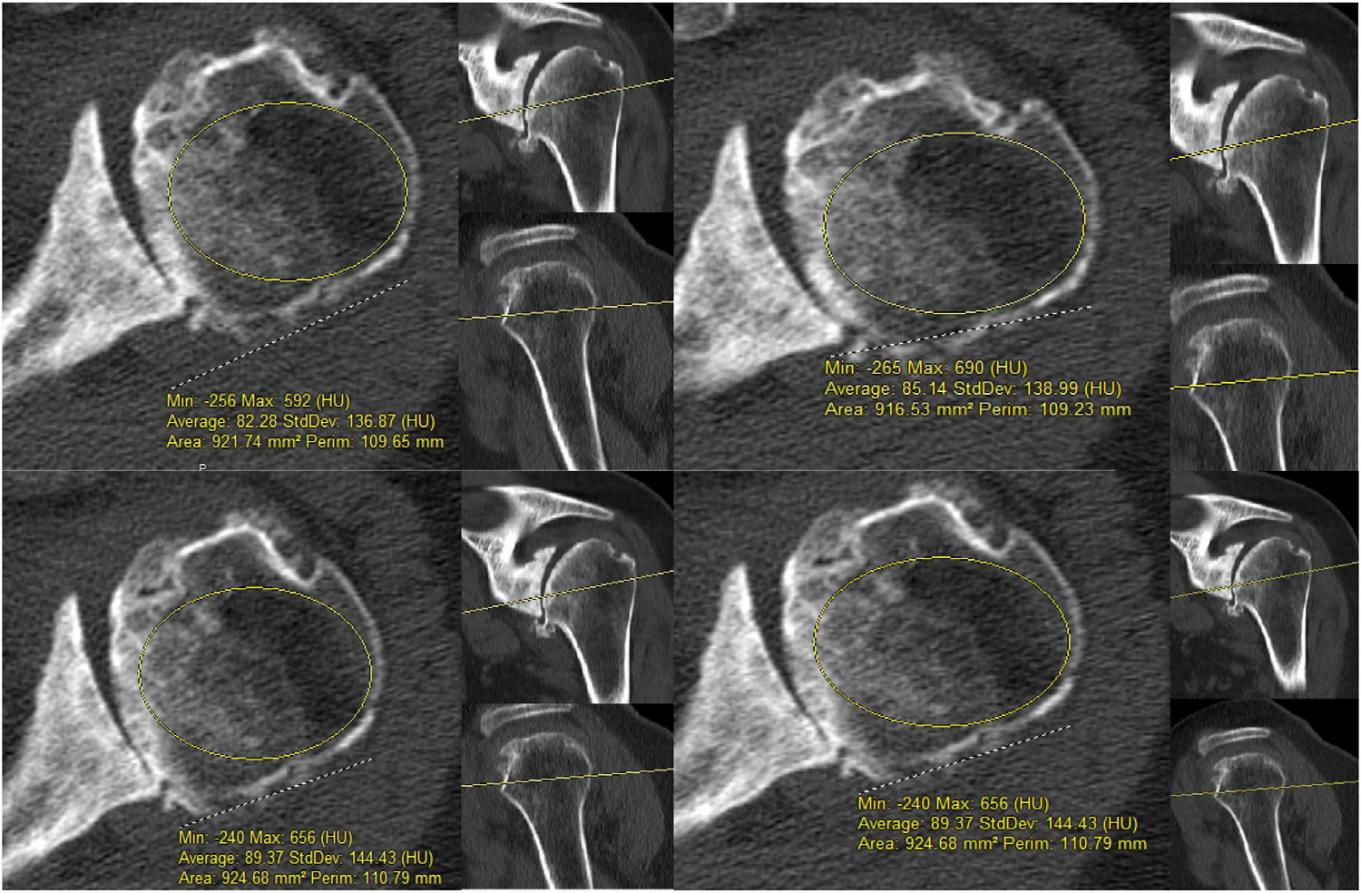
Computed tomography (CT) Hounsfield unit (HU) measurement. Four consecutive axial slices of the symptomatic shoulder CT scan were measured. A region of interest was drawn within the humeral head as outlined in this example. Average HU values were recorded. Two independent authors performed all measurements.
